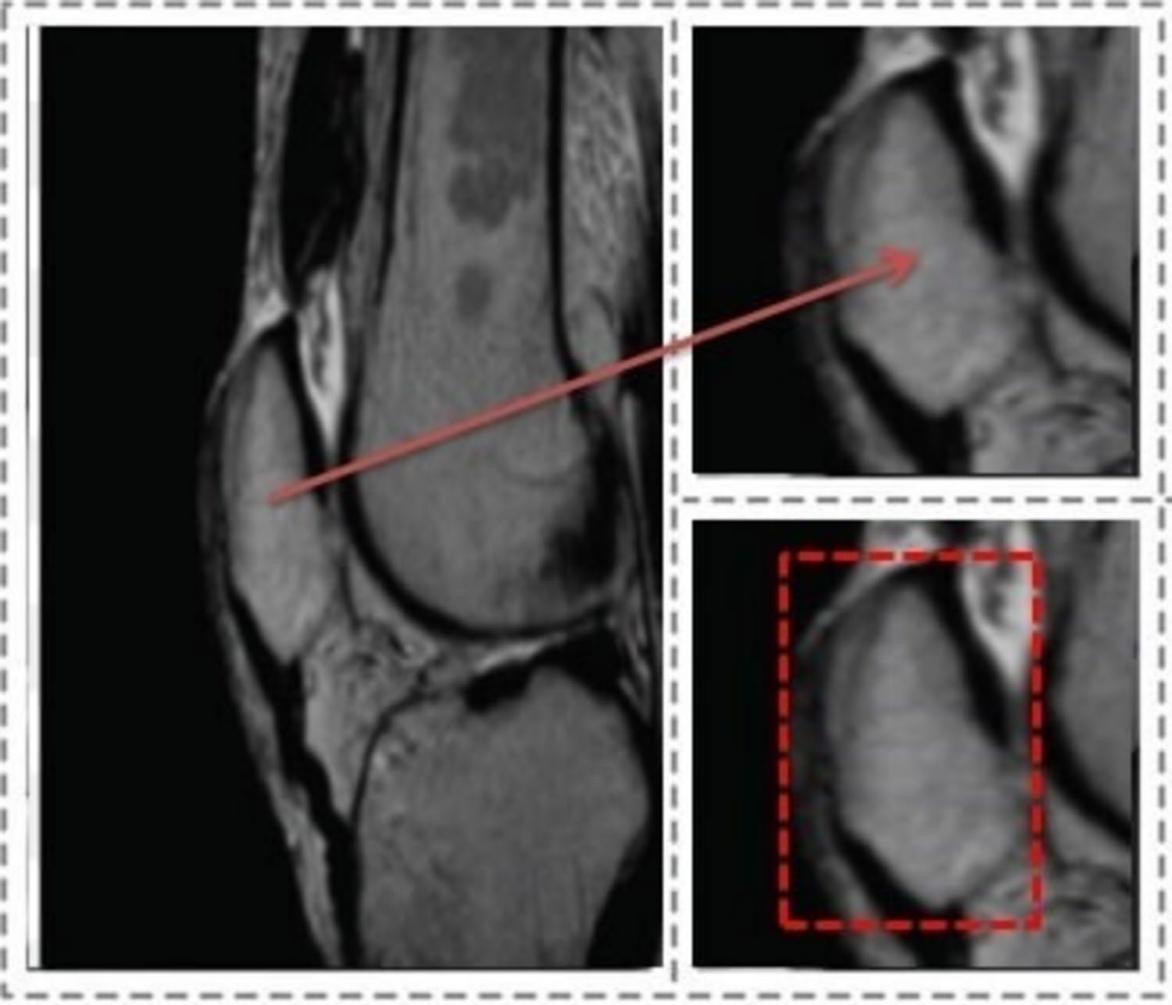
The patella MR image dataset
